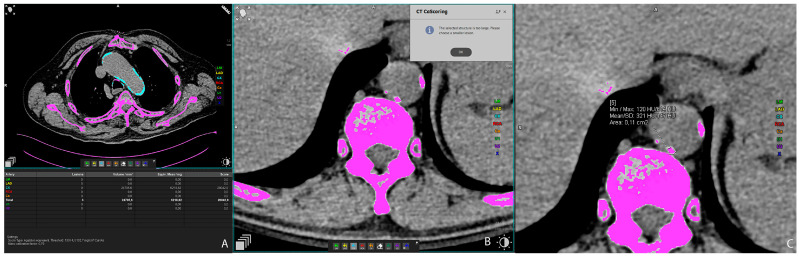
Representative axial CT images showing arterial calcification measurement using syngo.via postprocessing software VB60A_HF08 (A), and freehand ROI measurement of calcification volume (B,C).
